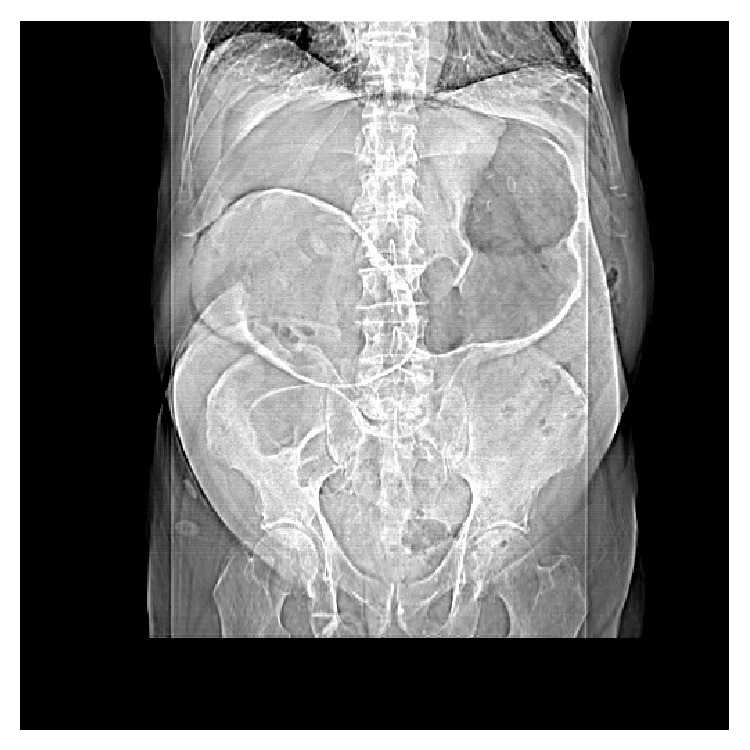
Abdominal X-ray revealing distended large bowel with a collapsed small bowel.
